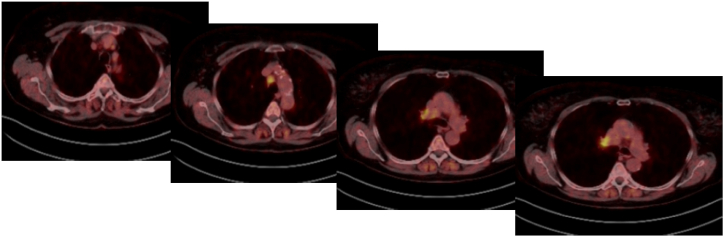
PET-CT-Scan after first two cycles of neoadjuvant chemoimmunotherapy: good response with reduced FDG-uptake.
